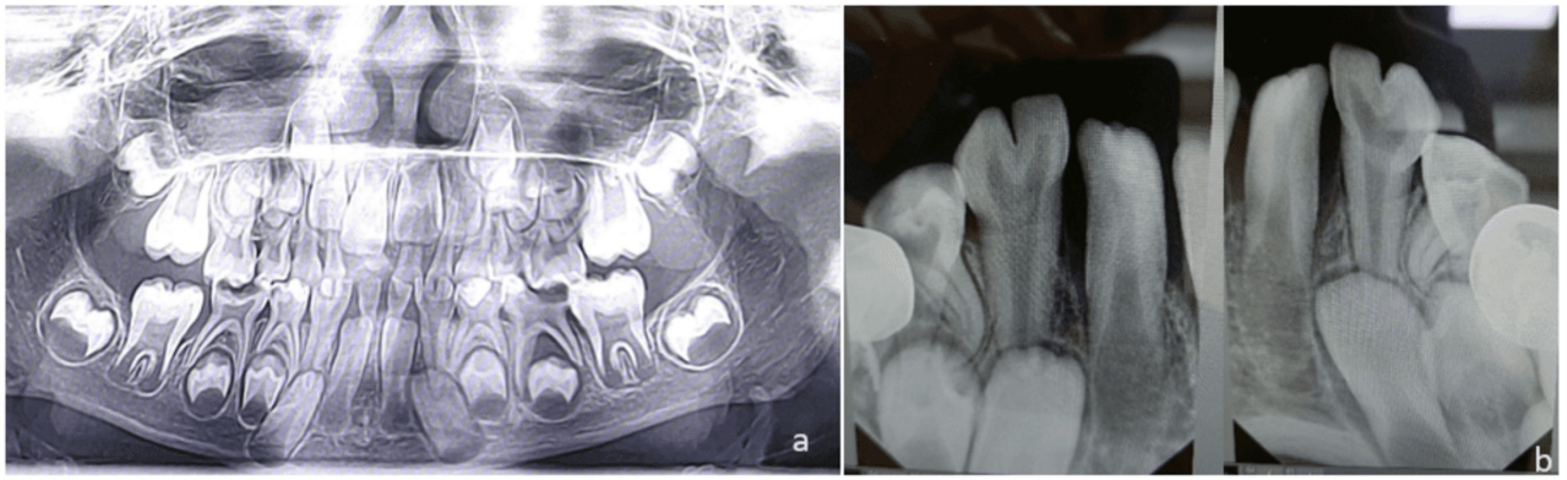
Case 1 radiographic images A - Orthopantomogram revealing fusion with respect to 72,73 and 82,83; Agenesis of tooth buds 32 and 42. B - Radiovisualgraph revealing type I fusion with respect to 72,72 and 82,83 with absence of tooth bud of lateral incisor bilaterally
